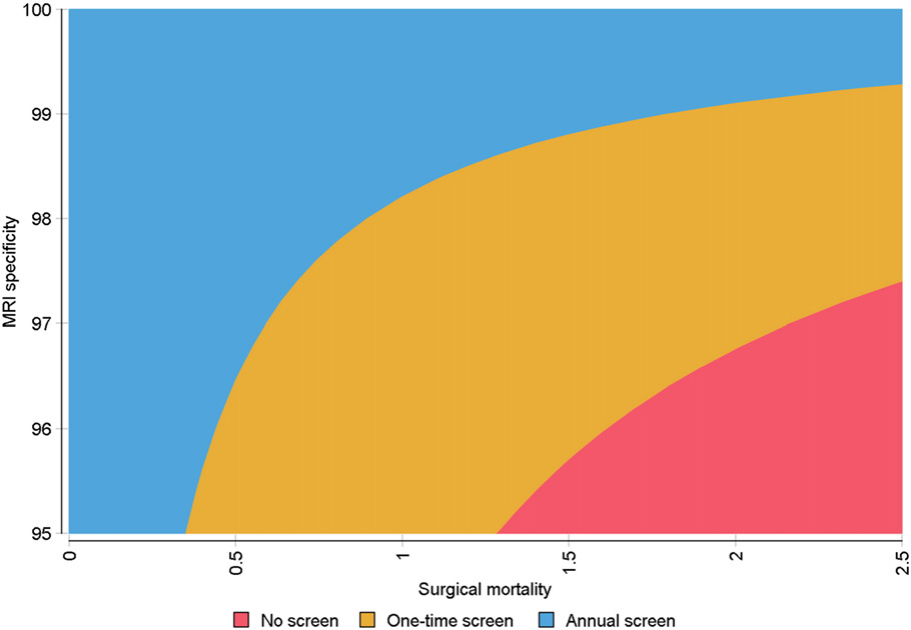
Two-way sensitivity analysis on effectiveness of screening in primary cohort BRCA2 carriers. Surgical mortality and MRI specificity were varied over ranges of 0–10% (0–2.5% graphed) and 50–100% (95–100% graphed), respectively.
